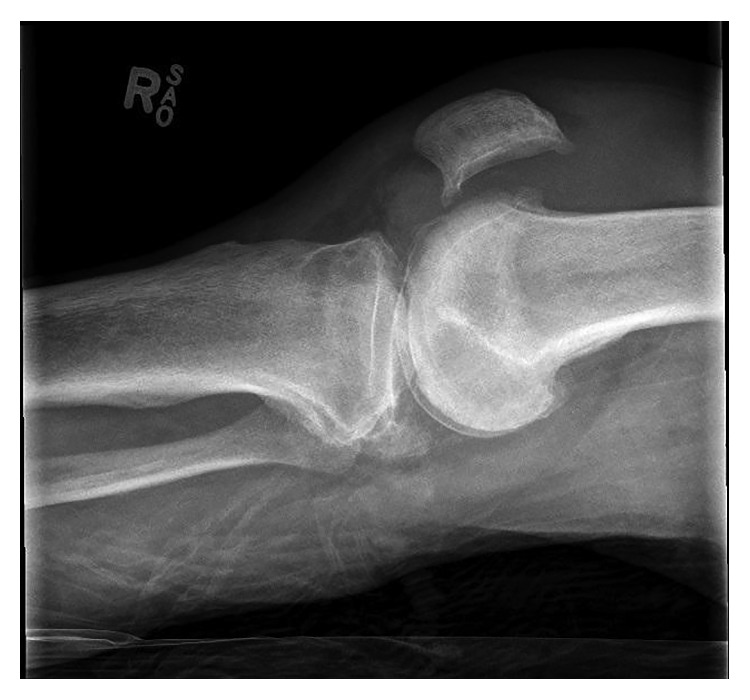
X-ray of the patient's right knee on the day of presentation shows soft tissue swelling but no discrete fracture. There is tricompartmental osteoarthritis with a moderately large joint effusion.
